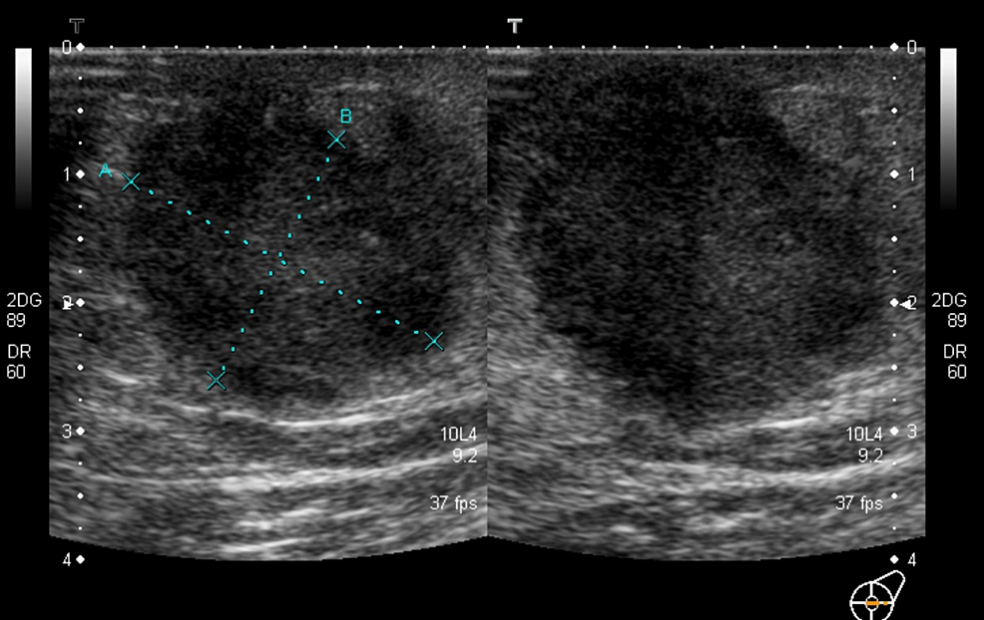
Ultrasound breast done as a part of the triple assessment The hypoechoic lobulated mass measured 27 x 21 mm with internal specks of calcification and increased posterior accentuation in the left retroareolar region. Imaging features were consistent with primary malignant breast lesion. No other lesions in the left breast were seen. Suspicious-appearing lymph nodes were seen in the left axilla, with the largest measuring 26 x 17 mm.
